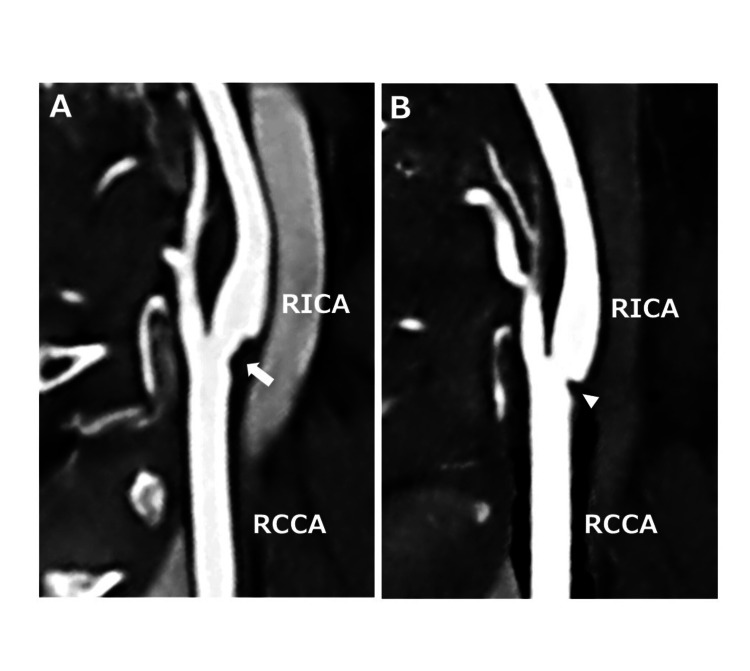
Three-dimensional CT angiography was performed after thrombectomy and one year before this admission. Sagittal multi-planar reconstruction of three-dimensional CT angiography performed after thrombectomy (A) reveals mild, insignificant (<50%) stenosis at the posterior wall of the right carotid bulb (arrow). Whereas, a shelf-like filling defect, suggestive of a carotid web, is recognized in three-dimensional CT angiography one year prior to this admission (B) (arrowhead). RCCA, right common carotid artery; RICA, right internal carotid artery
